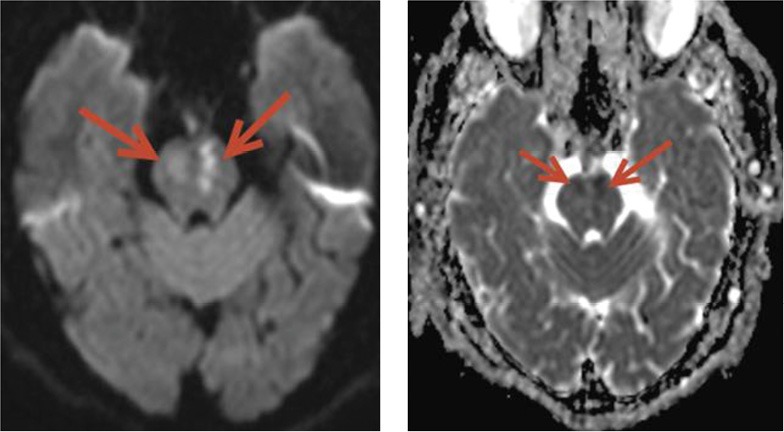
Brain MRI shows two areas affected by cerebral ischemia on the right and left part of the pons.
